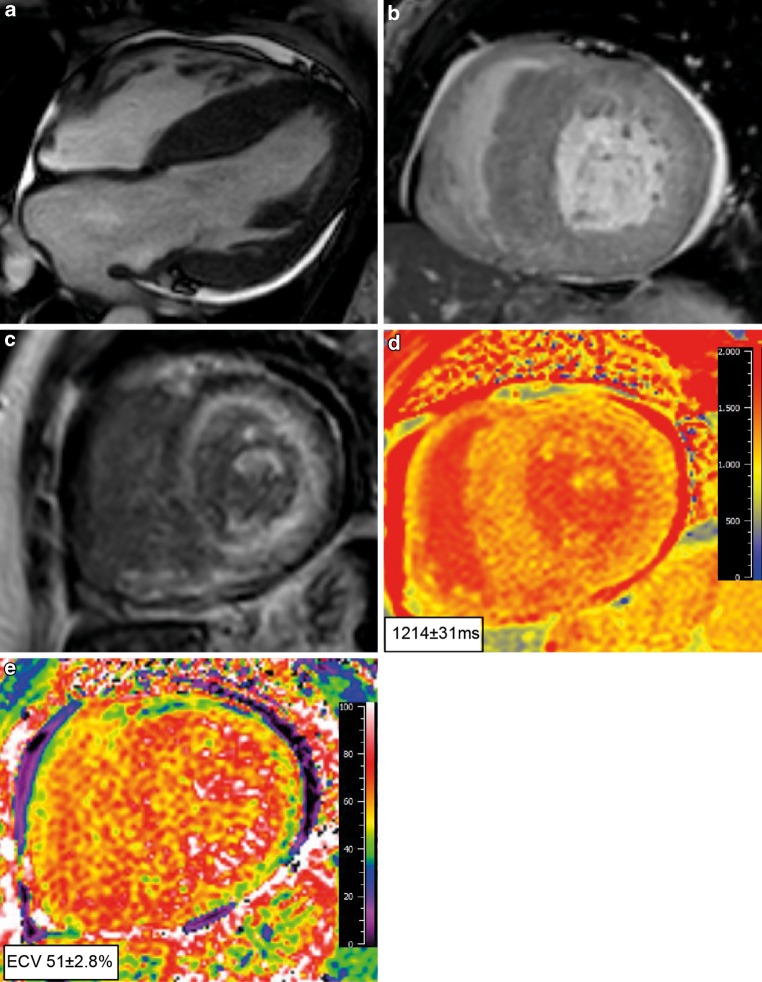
Cardiac magnetic resonance imaging in cardiac amyloidosis. a–b Cardiac magnetic resonance imaging showing a 4‐chamber and short axis view with left ventricular hypertrophy mainly in the septal region. Corresponding short axis view showing generalised subendocardial late gadolinium enhancement not fitting a coronary territory. Note the reduced signal of the blood pool (dark blood), which is specific for cardiac amyloidosis (c). Native T1 mapping analysis showing an increased T1 value of 1214 ± 31 ms (d) and subsequent ECV map which is increased giving a value of 51 ± 2.8% (e), both acquired with a field strength of 1.5 T
